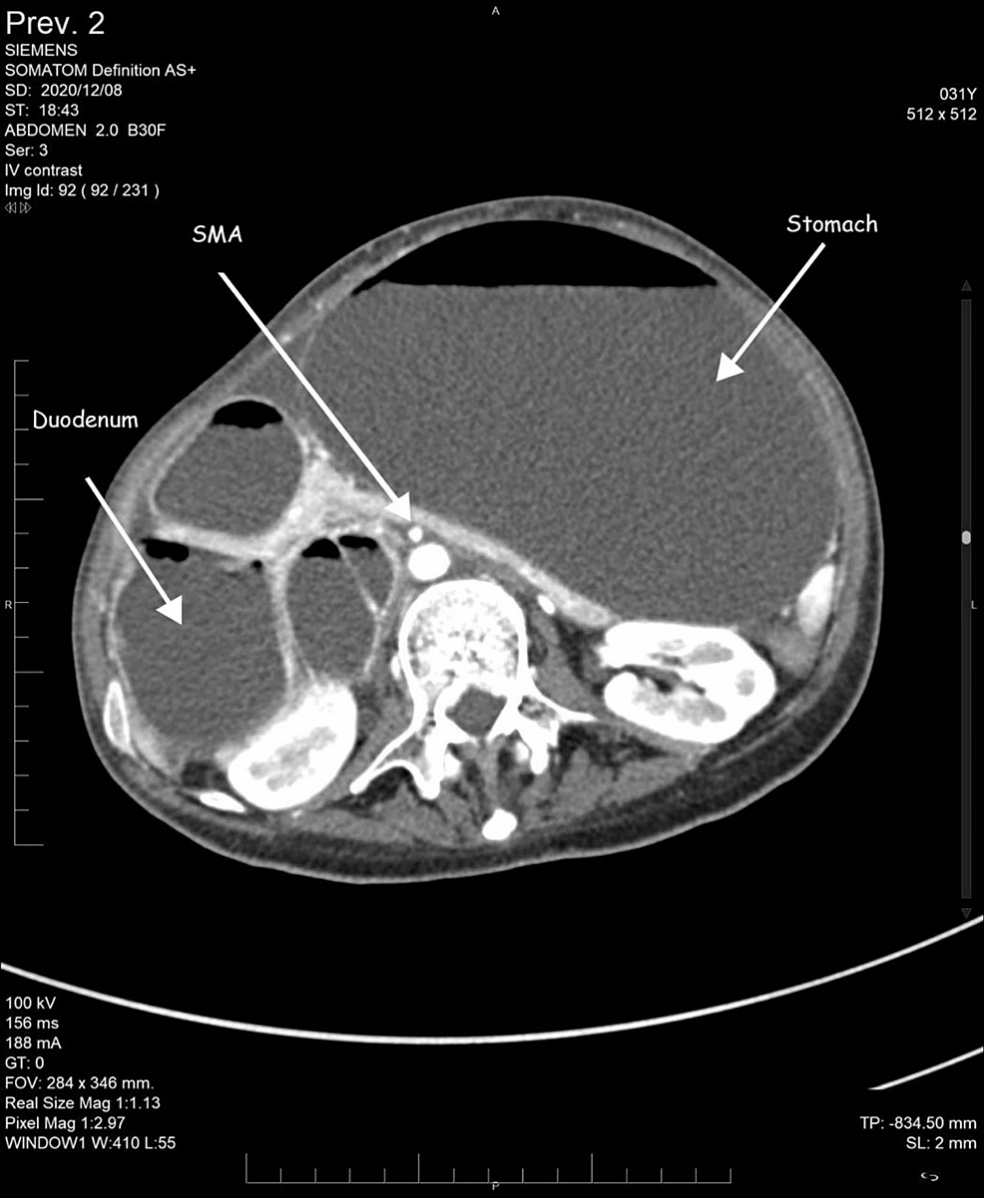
Axial contrast-enhanced CT of the abdomen showing a grossly dilated stomach and proximal duodenum. CT: Computed tomography; SMA: Superior mesenteric artery
